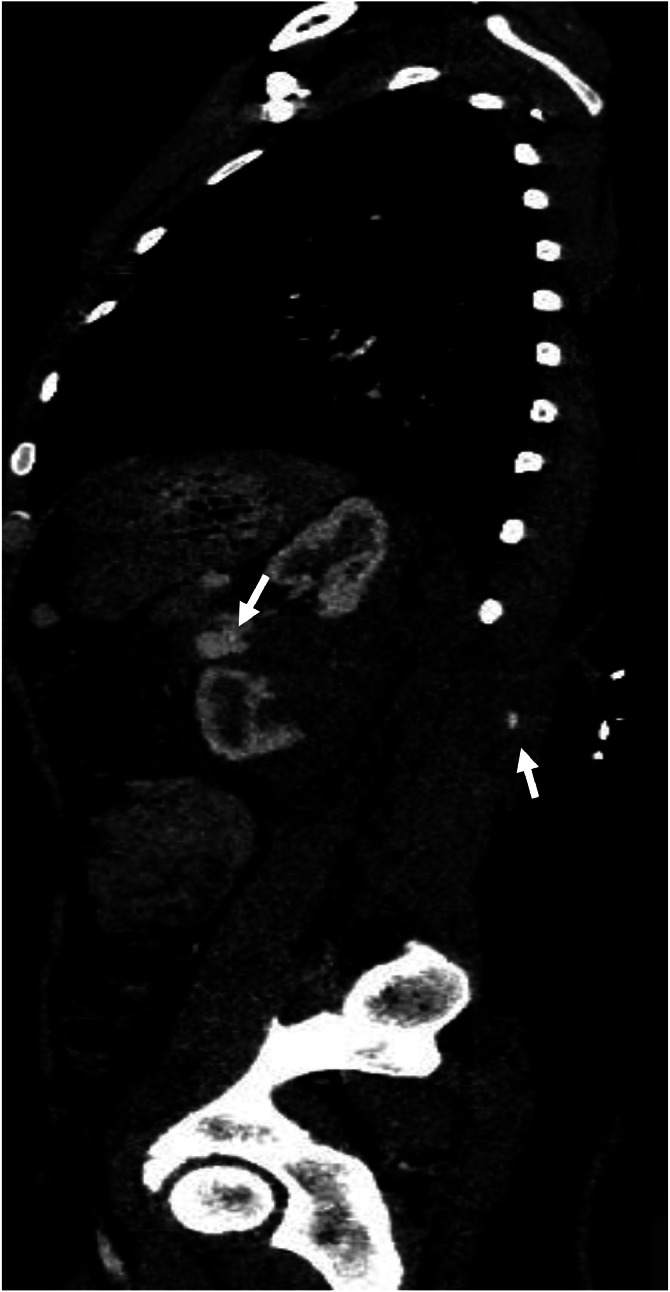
Sagittal contrast-enhanced abdominal CT showing a penetrating wound in the left lumbar region with an entry point adjacent to the L1 vertebra. The wound tract extends through the left longissimus thoracis muscle, associated with emphysematous bubbles and contrast extravasation along the trajectory.
